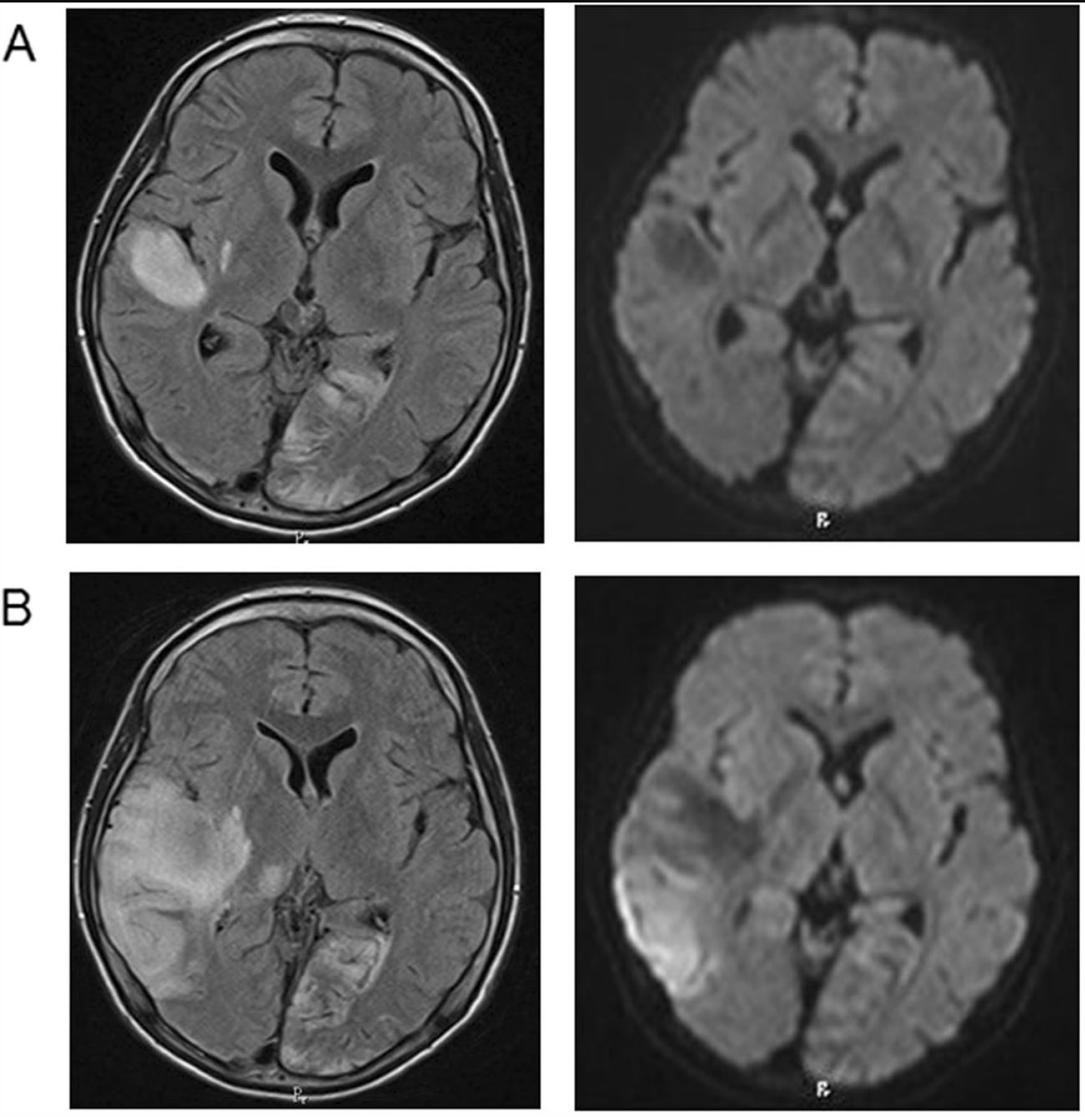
Sequential changes in MRI. (a) Brain MRI on admission shows small multifocal gray matter regions in the right temporal, parietal, and occipital lobes, and diffuse white matter lesions in the left temporal lobe, visualized as high-intensity areas in FLAIR images (left). White matter lesions in the left temporal lobe show low intensity in diffusion-weighted images (right). b Extended white matter and left thalamic lesions appeared after a stroke-like episode
